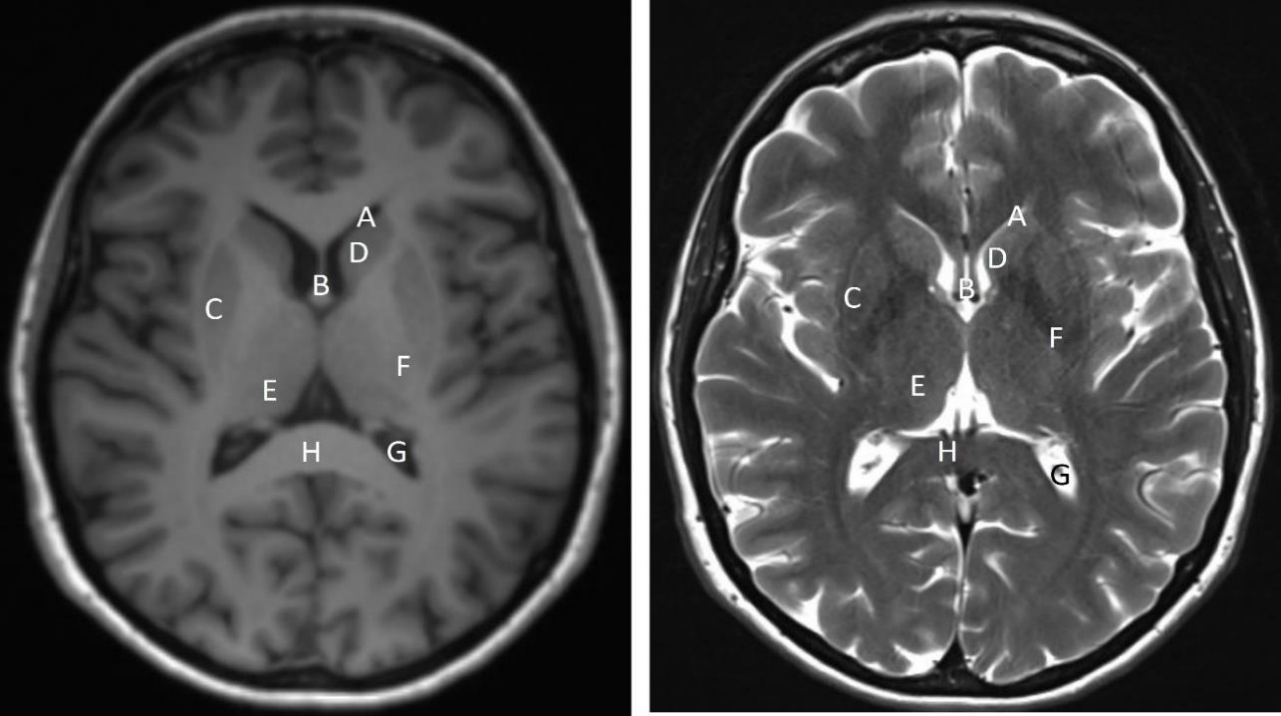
T1 (left) and T2 (right) weighted axial MR images of the brain of a 45 years-old female at the level of the basal ganglia shows normal anatomy. Cerebrospinal fluid (and fluid elsewhere in body) appears hypointense (black) on T1 and hyperintense (white) on T2 weighted images respectively. Fat appears hyperitense on both T1 and T2 weighted images. It is to be noted that signal intensity of a given structure changes on various sequences used during MR imaging
